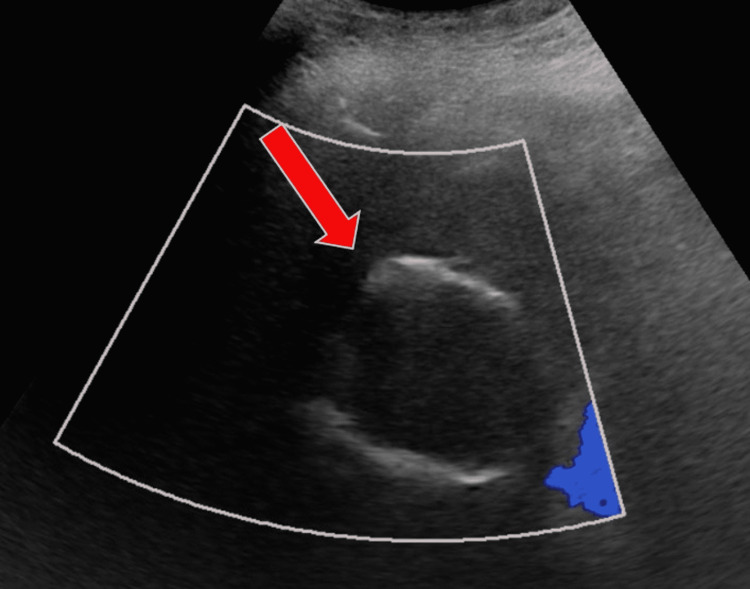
Ultrasound with color doppler along the hepatic dome demonstrates a peripherally echogenic round avascular mass (red arrow), which is centrally obscured by the echogenic walls.
